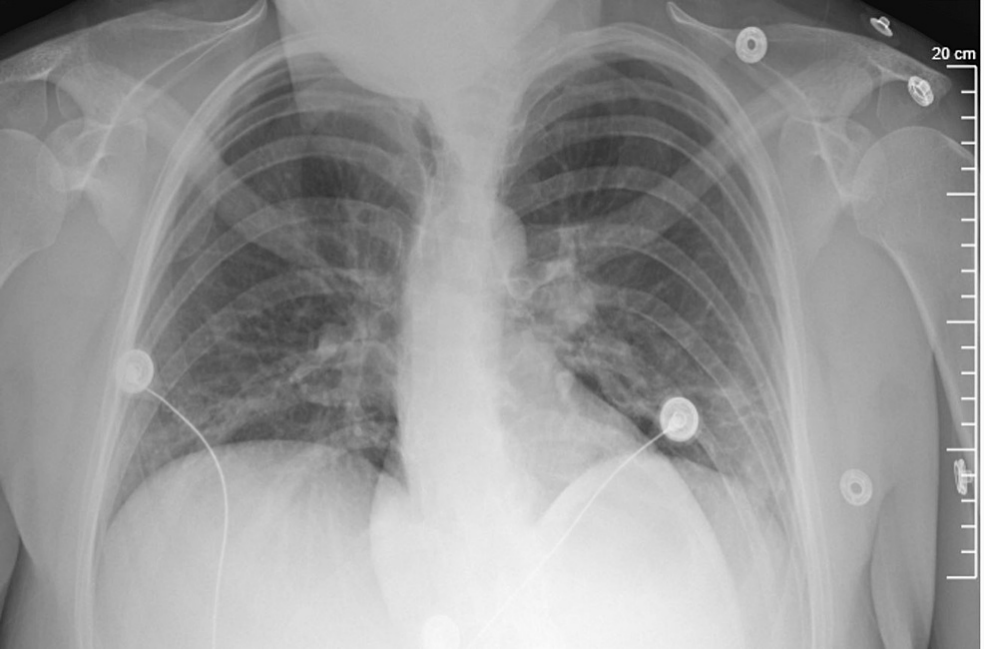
Anterior posterior radiograph of patient post operation. Nodule was removed in its entirety.
